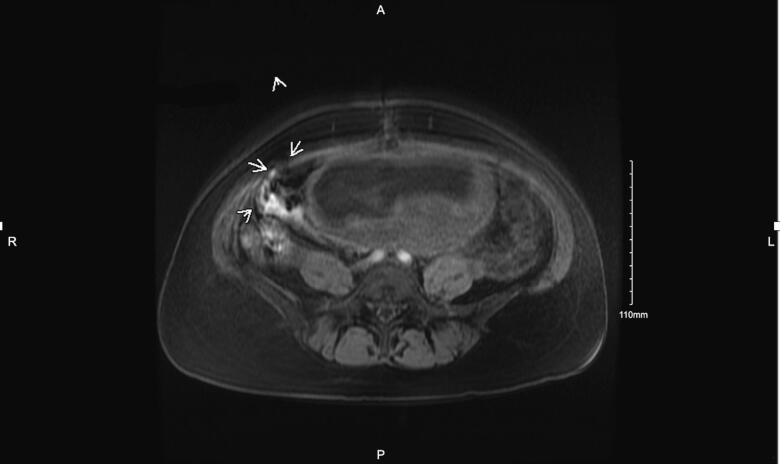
Magnetic resonance imaging (MRI) of abdomen after initial fertility-sparing staging surgery showing a soft tissue implant in the right lower quadrant, consistent with residual disease.
